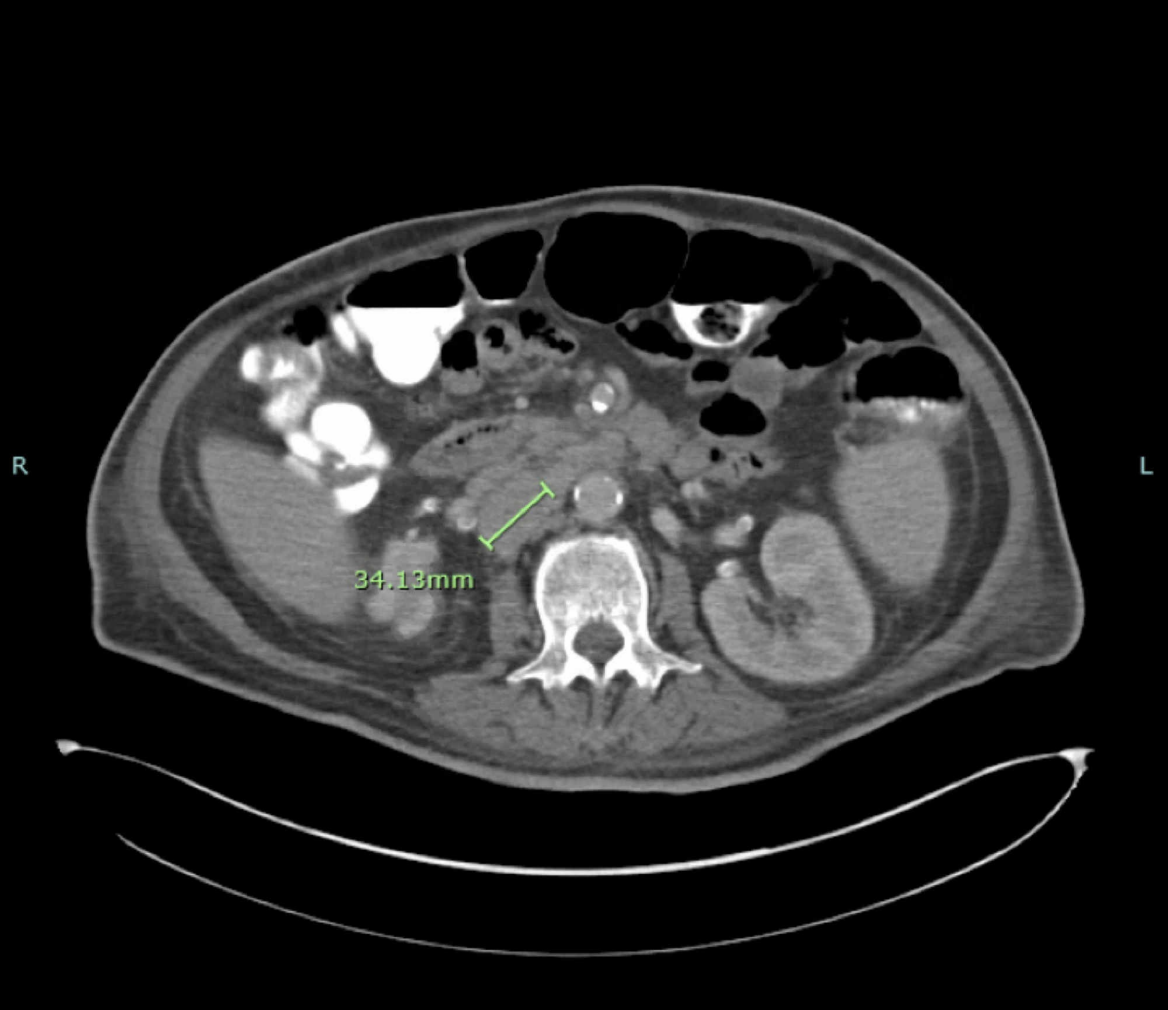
CT of the abdomen and pelvis demonstrating extensive retroperitoneal lymphadenopathy. Outlined in green is the largest node in the retrocaval area measuring 3.4 cm
